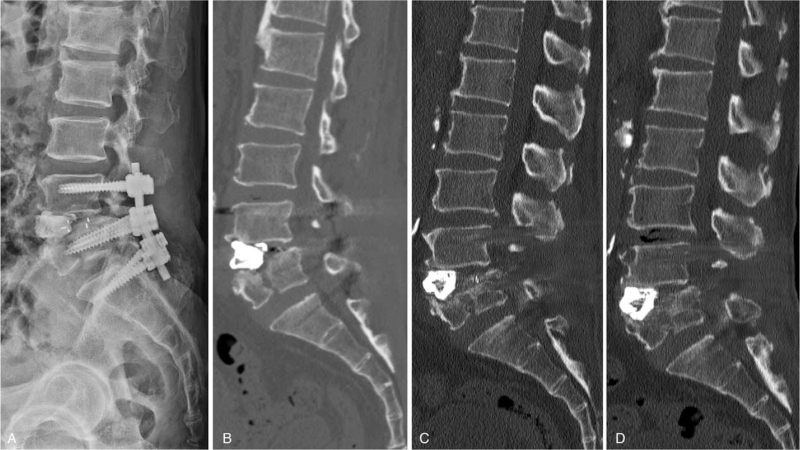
Serial follow-up postoperative imaging. (A) Postoperative lateral lumbar radiography showed posterior lumbar interbody fusion L4-5 and the screw extended to S1 with interbody cement augmentation. (B) CT after 6-months follow-up showing that the bone fragment is not fused. (C) CT after a 1-year follow-up showing an interbody space and bone fragment fused partially. (D) CT after a 2-years follow-up showing interbody space and bone fragment fused solid. CT = computed tomography.
